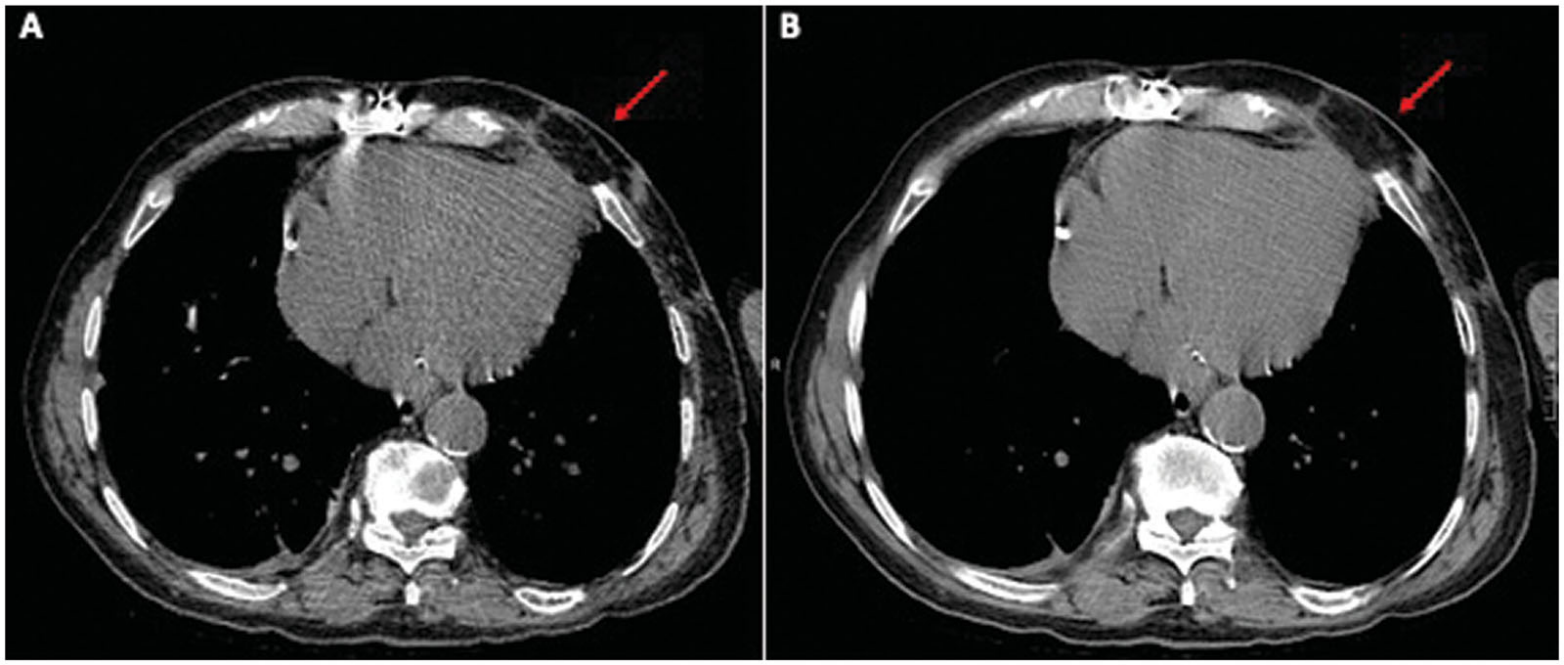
A CT scan executed 1 month post-op (A) and 1 year post-op (B) clearly shows the trophism of the flap (red arrow) and no collection.
